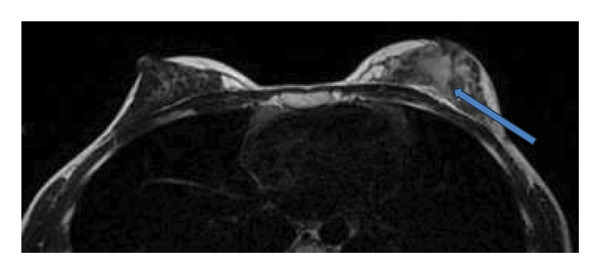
The same patient as in Figure 5. MRI T2‐weighted sequence demonstrates diffuse edema of the left breast parenchyma, with some internal fluid component extending to the inverted nipple. On postcontrast imaging, there is a diffuse increased enhancement of the entire left breast parenchyma in the ce‐T1 sequence, with an abscess‐like formation in continuity with the lactiferous duct and associated with nipple inversion. The patient recovered after intravenous antibiotic therapy with clindamycin.
